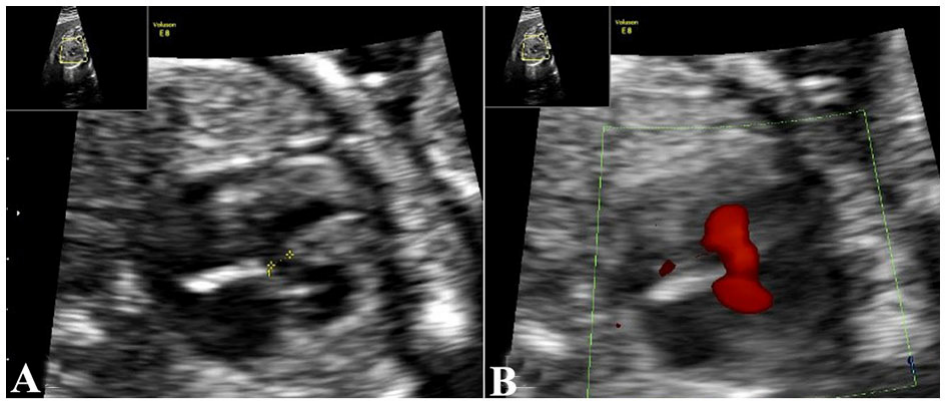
Echocardiography demonstrates cardiac malformations. (A) Echocardiography demonstrates a 0.21-cm interruption of continuity in the ventricular septal membrane. (B) trans-septal blood flow signals at the VSD. VSD, ventricular septal defect.
